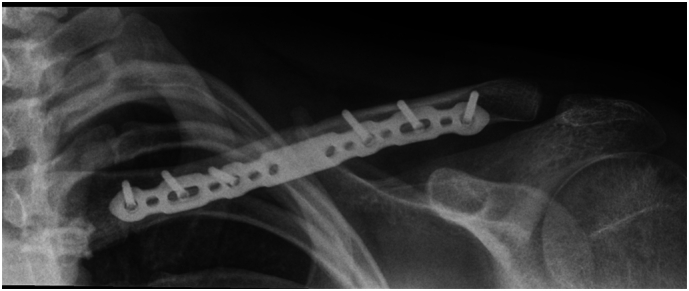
Radiograph of left clavicle at 3 months post operation, showing satisfactory alignment and position of left clavicle.
